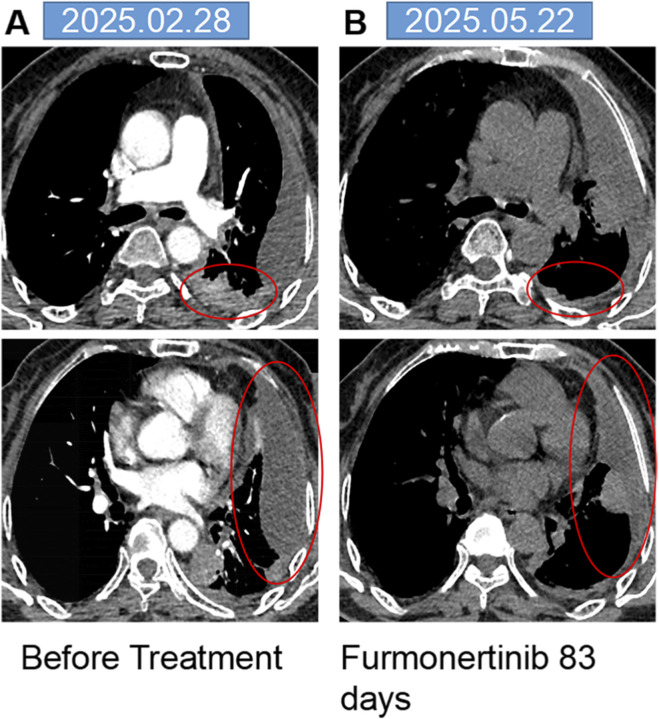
The mediastinal window of the chest CT. (A) Chest CT showed the lesions in the left lung as indicated within the red circle. (B) After 83 days of furmonertinib treatment, the area within the red circle showed that the lesions had reduced in size compared to before.
